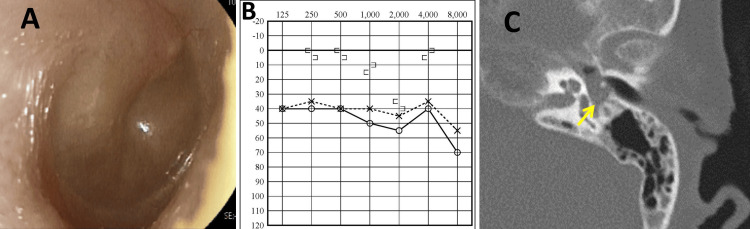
Ear findings prior to tezepelumab treatment. (A) Otoscopic findings before tezepelumab treatment: Swelling of the bilateral posterior superior quadrant of the tympanic membrane and middle ear effusions were observed. (B) Pure tone audiometry before tezepelumab treatment: Pure tone audiometry revealed a conductive hearing loss. (C) Temporal bone CT scan showing soft tissue shadows in the tympanic cavity before treatment (yellow arrow).
